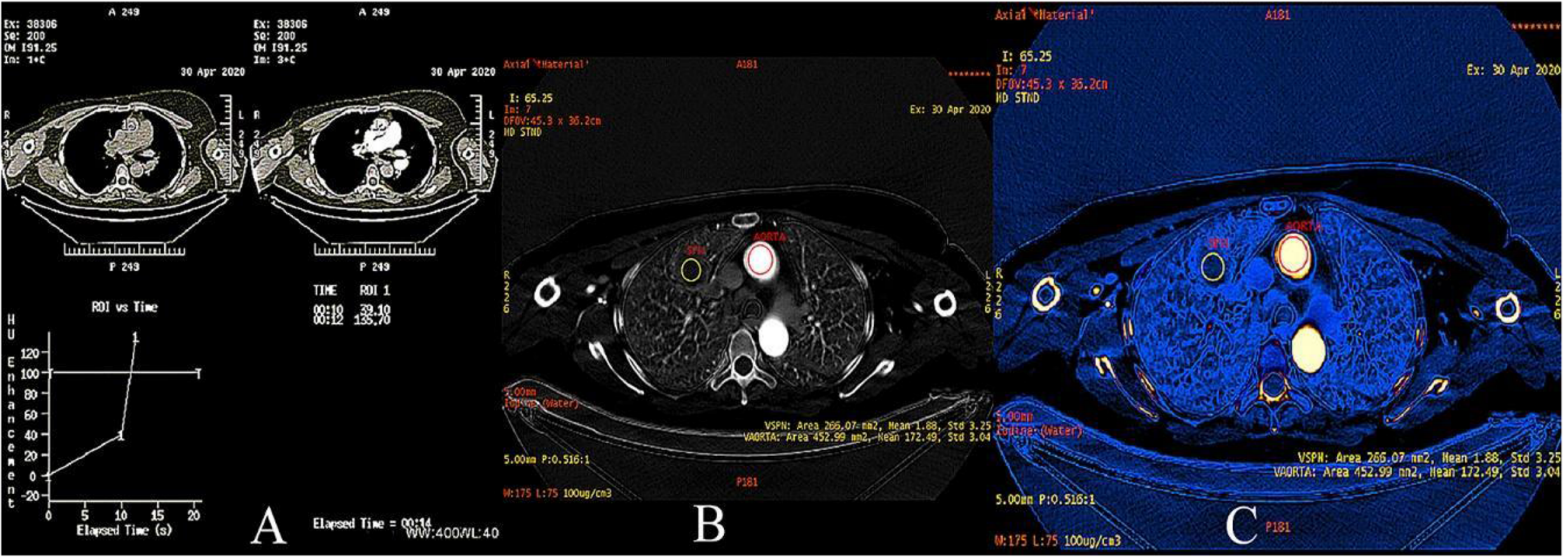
Exceeding the density of 100 HU in the ROI in the ascending aorta initiates the DECT (A) scan*.* Presentation of iodine concentrations at the reference site on the Linear Gray map (B) scan and the Color map (C) scan. DECT (ascending aorta—red ROI) and in the lung tumor (SPN—yellow ROI). Iodine concentration in the aorta and lung focal lesion is 172.49 × 100 µg/cm^3^ and 1.88 × 100 µg/cm^3^, respectively. *DECT* dual-energy computed tomography, *SPN* solitary pulmonary nodule, *ROI* region of interest.
